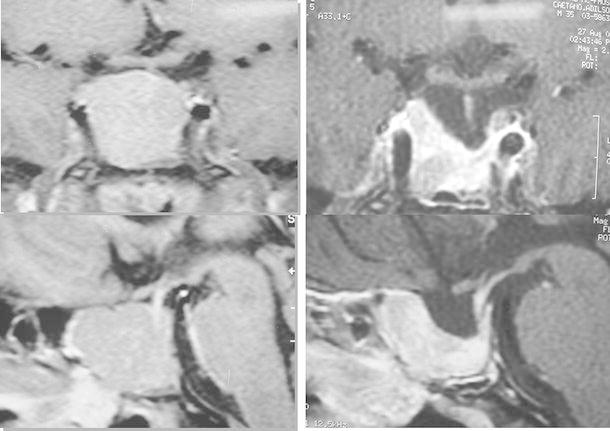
MRI scans of pituitary tumor at diagnosis (April 2008, left) and following non-curative surgery (January 2009, right) prior to commencing pharmacological therapy
